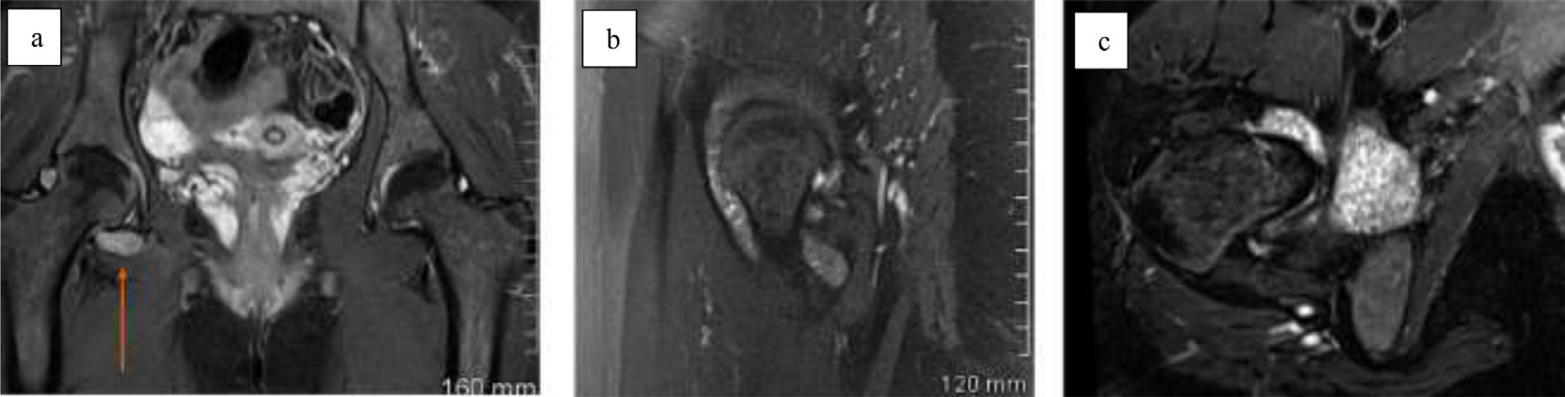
Case 2. Pelvic MRI sequences in a 26-year-old female. MRI demonstrates a moderate right hip joint effusion with multiple hypointense intra-articular bodies. (a) Coronal STiR sequence, (b) sagittal PD fat-suppressed sequence of the right hip, (c) axial T2 fat-suppressed sequence of the right hip.
